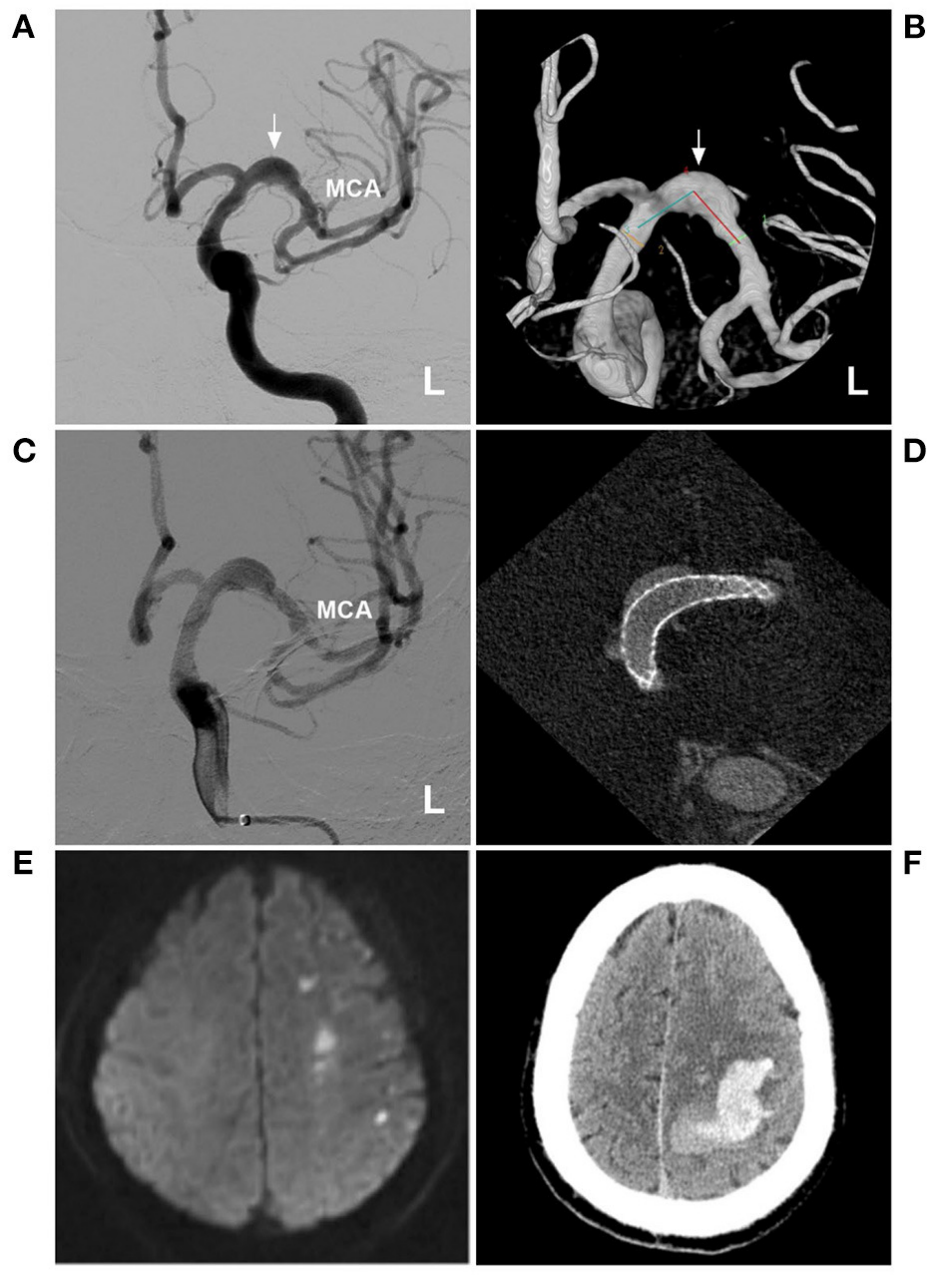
FD deployment for a fusiform aneurysm of the proximal MCA. (A,B) DSA (A) and three-dimensional DSA (B) showed a fusiform aneurysm of the proximal MCA (arrows). (C,D) DSA (C) and maximum-intensity projection image (D) showed FD deployment. (E) MRI showed acute infarction in the frontal and parietal lobes. (F) CT showed the delayed intracerebral hemorrhage. CT, computed tomography; DSA, digital subtraction angiography; FD, flow diversion; MCA, middle cerebral artery; L, left; MRI, magnetic resonance imaging.
